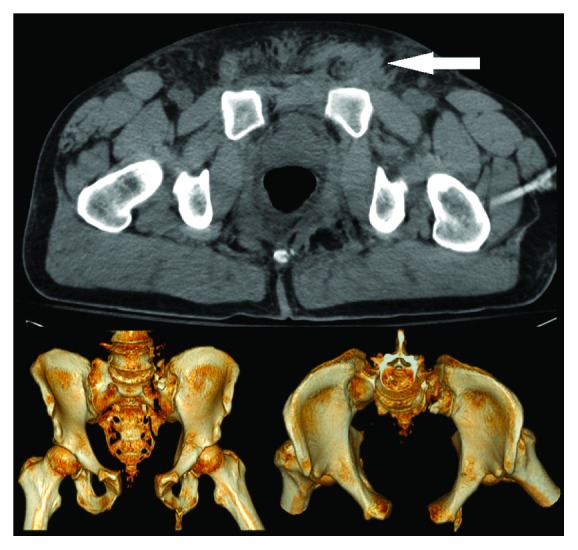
CT Reconstruction demonstrating open-book pelvic fracture. The white arrow demonstrates the inguinal hernia.
